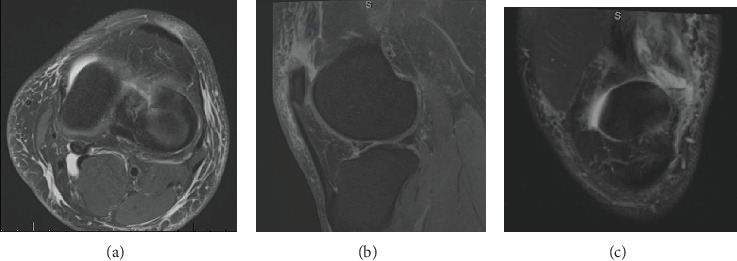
(a) Axial, (b) sagittal, and (c) coronal T2 MRI views showing isolated vastus lateralis tendon rupture with approximately 3 cm proximal retraction.
